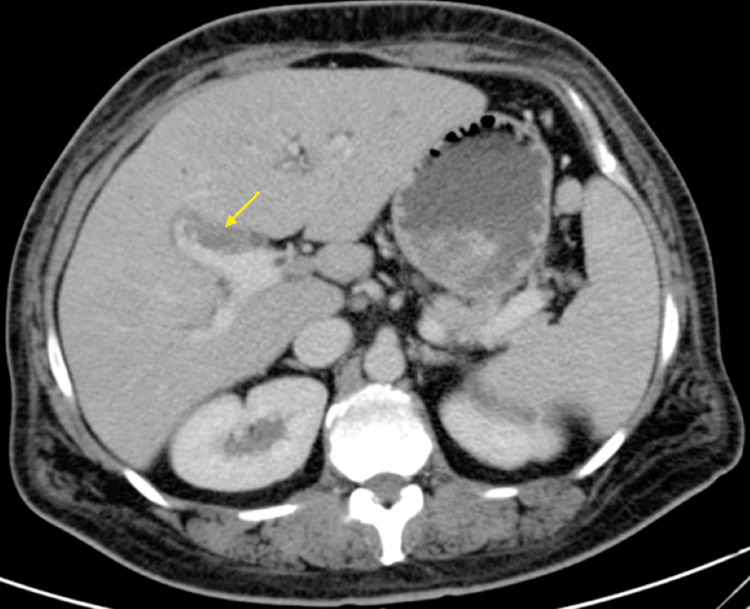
CT abdomen and pelvis with intravenous contrast showing intra-hepatic biliary dilatation and filling defect at the level of the CHD extending to the right hepatic duct. CT: computed tomography, CHD: common hepatic duct
